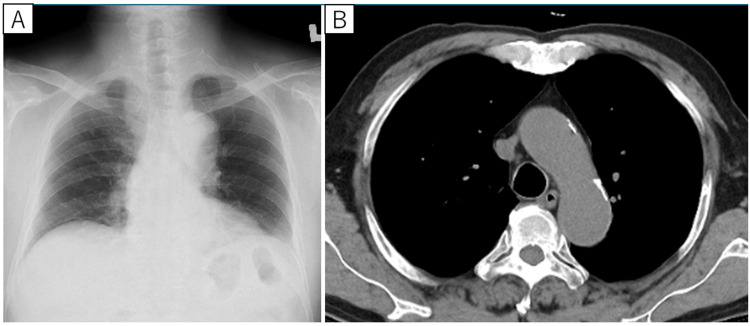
Postoperative chest imaging performed by the Department of Surgery following appendiceal cancer resection, with no respiratory abnormalities noted at that time A postoperative chest X-ray obtained six years prior to symptom onset (A) showed no mediastinal enlargement. Similarly, a chest CT scan performed one year and eight months before symptom onset (B) revealed no apparent abnormalities, including lymph node enlargement CT: computed tomography
